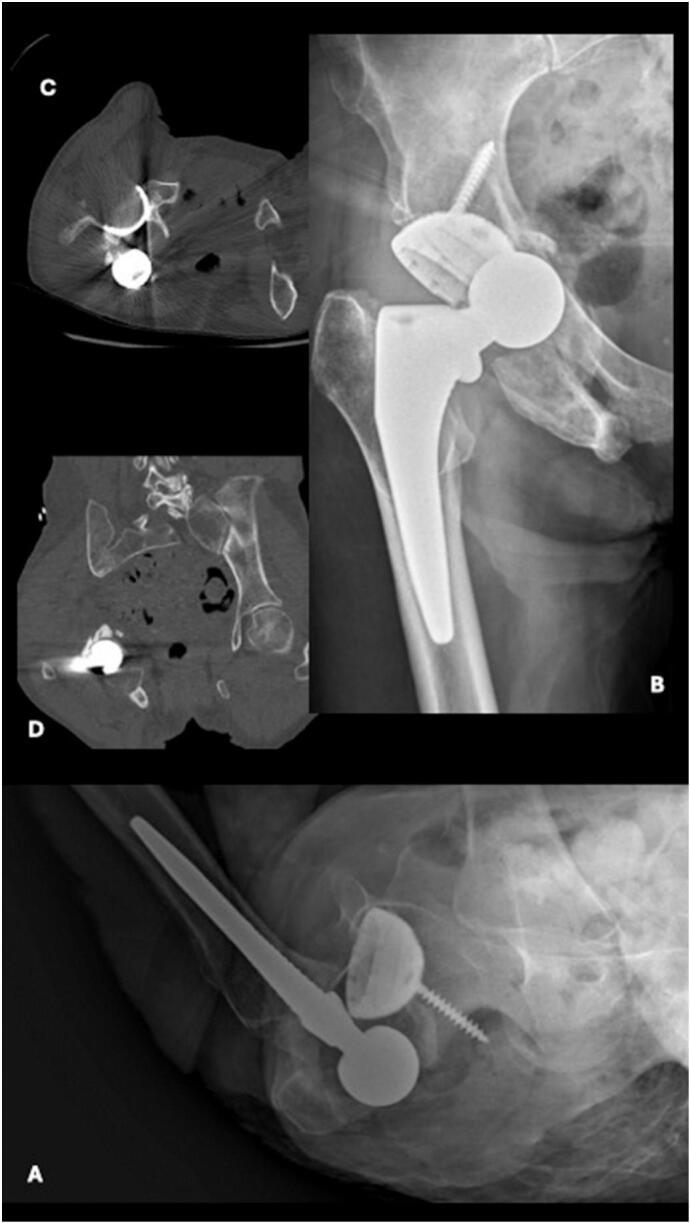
AP (A) and lateral (B) radiographic images of right hip showing right total hip arthroplasty with inferomedial dislocation and associated ischial and inferior pubic ramus fractures. Axial (C) and Coronal (D) CT images of right hip showing right total hip arthroplasty with inferomedial dislocation.
